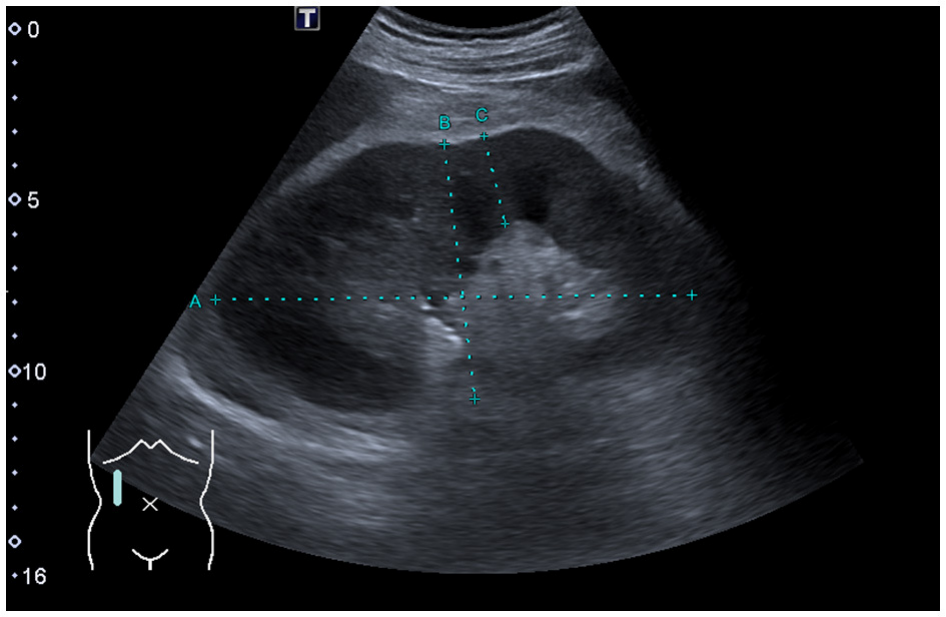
Renal ultrasound findings. Sagittal view of the right kidney showing a normal-sized kidney with swollen parenchyma measuring 26 mm (ref. range: 13–18 mm).
